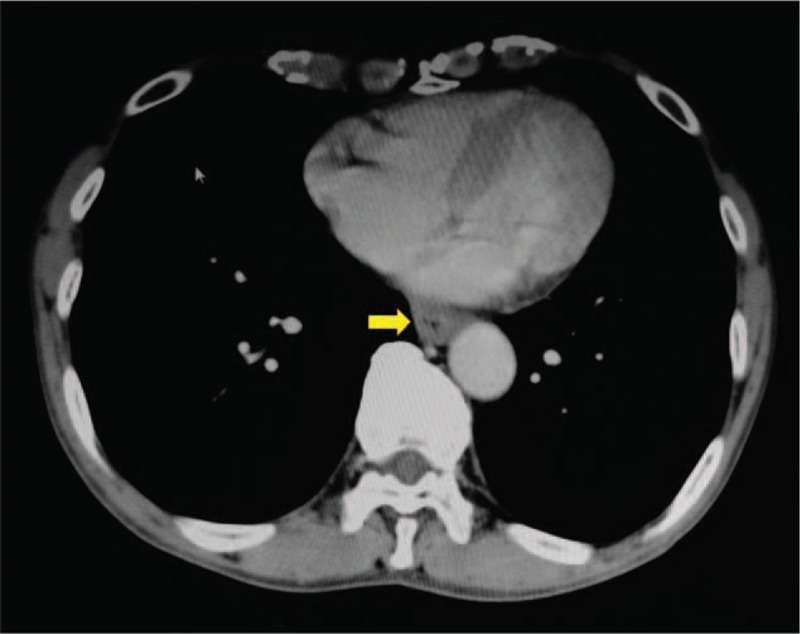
A contrast enhanced computed tomographic image of the thoracic organs demonstrates no remarkable changes, except for slight thickening of the wall in the lower thoracic portion of the esophagus (arrow).
